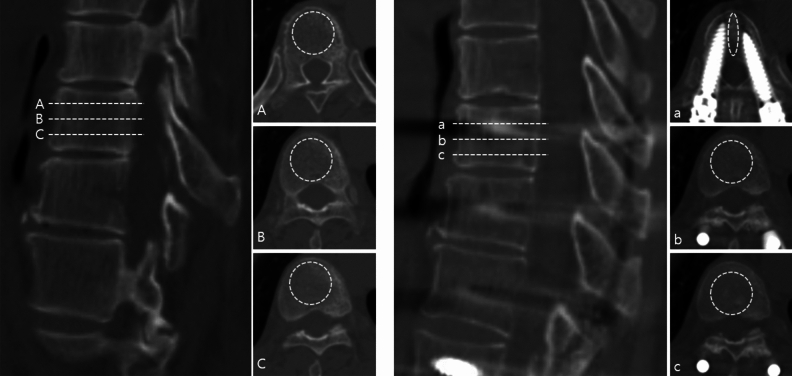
Measurement of Hounsfield Units (HU) from computed tomography using the Scheriber measurement methods. HU was measured within a circular range in the axial view in three sections divided by height. The HU of the vertebral body was defined as the average HU value of the three sections. In the vertebral body where the pedicle screw was inserted, HU was measured in an oval shape in the space between the pedicle screws. Afterwards, the process is the same.
